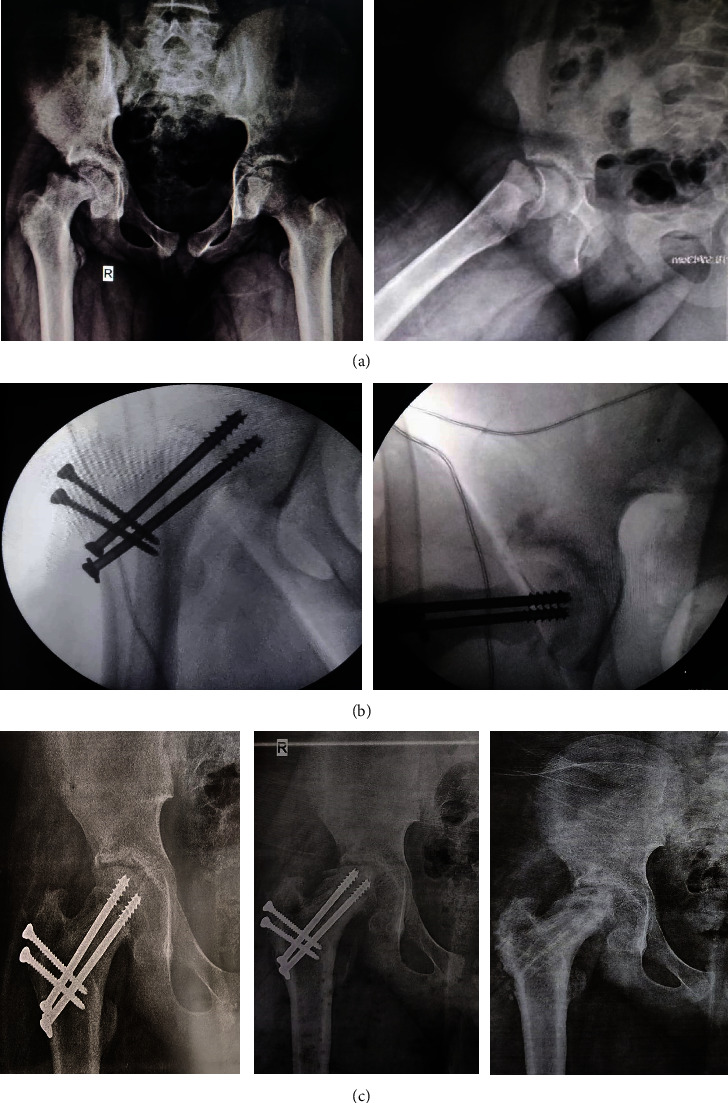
Radiographs of a 14-year-old male patient with right unstable severe SCFE. (a) Preoperative. (b) Intraoperative showing anatomical reduction. (c) Postoperative AP images at 3, 6, and 12 months follow-up showing signs of AVN starting at 3-month mark.
